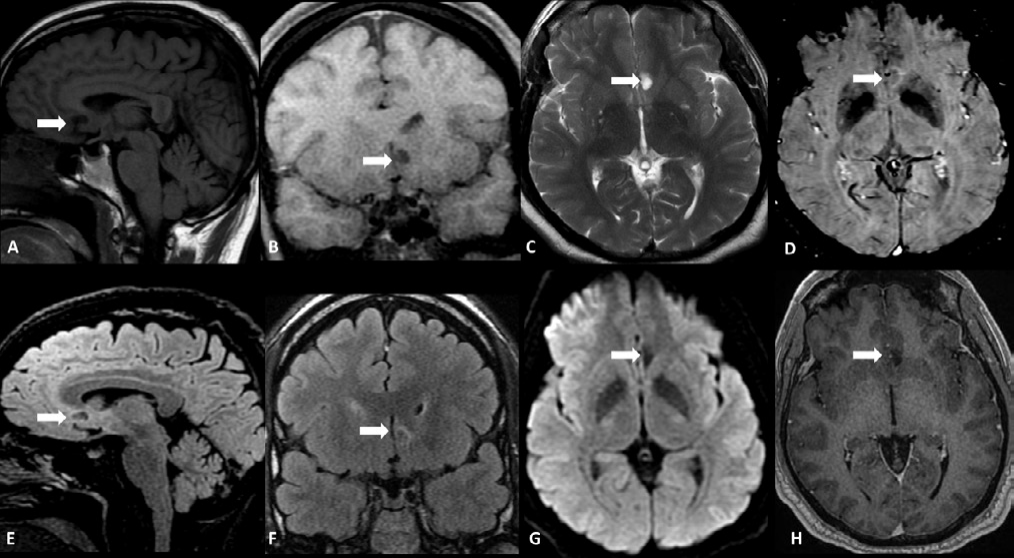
A 59-year-old female with chronic headache. Conventional MRI shows a round-shaped infiltrative lesion in the left septal/subcallosal area (white arrow) with a hypointense signal on Sagittal (A) and Coronal (B) T1W and hyperintense signal on T2WI (C). Note the central hypointense and peripheral hyperintense signals on both SWI (D), Sagittal (E) and Coronal (F) FLAIR. The lesion demonstrates facilitated diffusion on DWI (G), and no contrast-enhancement on Axial CE-T1WI is seen (H).
